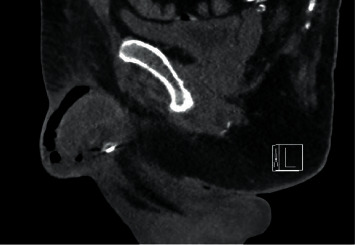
Computed tomography (CT) of the abdomen and pelvis: significant infiltration of the whole of the penis until its origin and its periphery at the pubic level, with many air bubbles within it. No infiltration of the perineum. Diffuse calcified atheromatous. Fournier gangrene located at the penis level.
